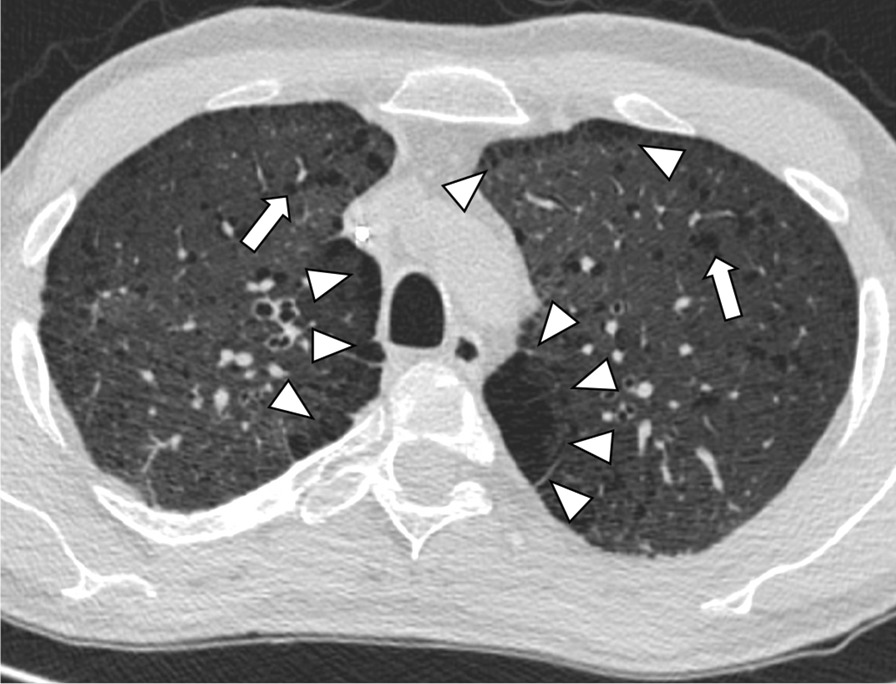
Transversal CT image of a 43-year old male smoker with extensive paraseptal emphysema in both apical upper lobes (white arrowheads) and multiple bilateral pulmonary cysts (white arrows)
